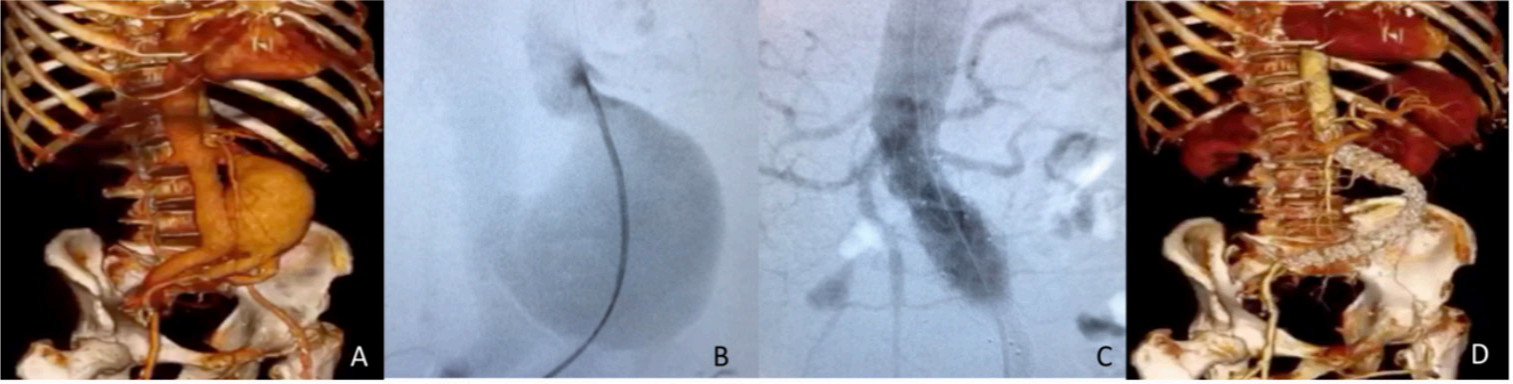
Case#1 (A) Preoperative 3D-CTA showing the RAAA involving an AF, demonstrated by early filling of the inferior vena cava; (B) Intraoperative DSA; (C) Intraoperative DSA after endograft deployment; (D) Postoperative 3D-CTA showing complete AF resolution. AF = Aortocaval fistula; DSA = digital subtraction angiography; RAAA = ruptured abdominal aortic aneurysms; 3D-CTA = three-dimensional-by-volume CT angiography.
